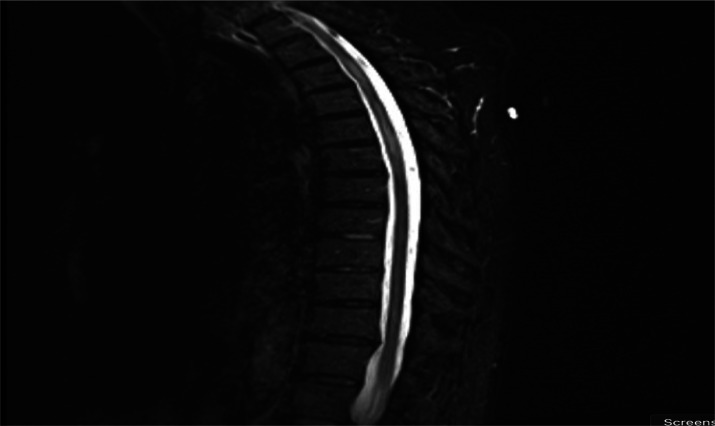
MRI of the thoracic spine in saggital view - T4-7 non-enhancing intramedullary lesion suggestive of transverse myelitis.
